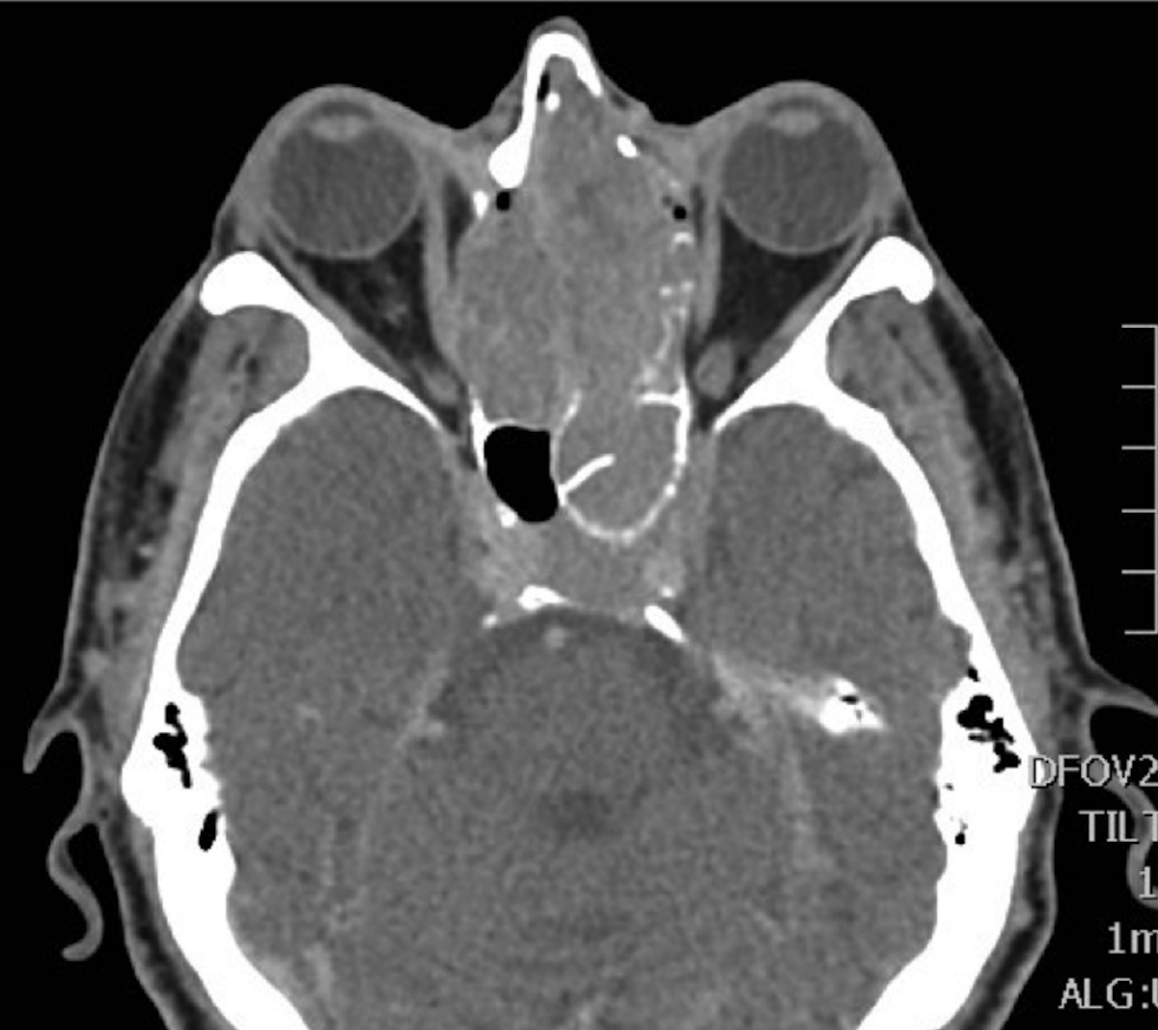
Contrast-enhanced computed tomography scan Axial cut showing the lesion extending into the left sphenoid sinus, involving the cavernous sinus and engulfing the left internal carotid artery.
